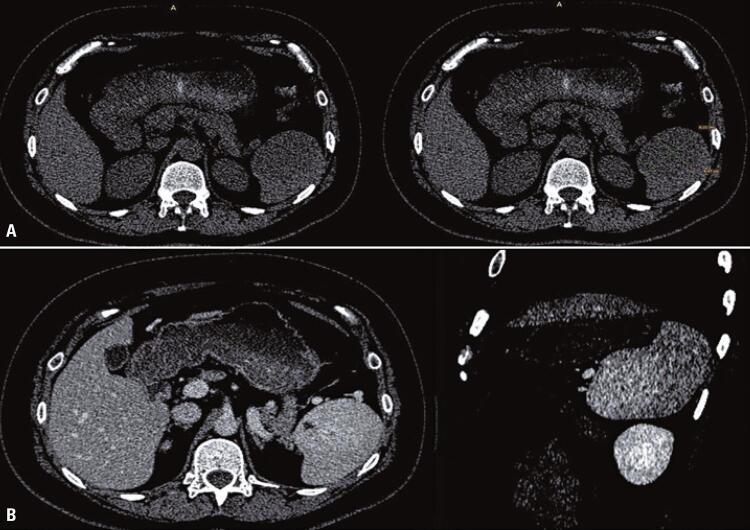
Computed tomography (without contrast) - Axial plane: oval, regular contoured, hypoattenuating mass located on the inferior and lateral aspect of the spleen (A). Computed tomography - Axial and sagittal plane: hypervascular enhancement about the adjacent splenic parenchyma, predominantly homogenous, presenting sparse hypovascular foci, without signs of extracapsular extension (B)
